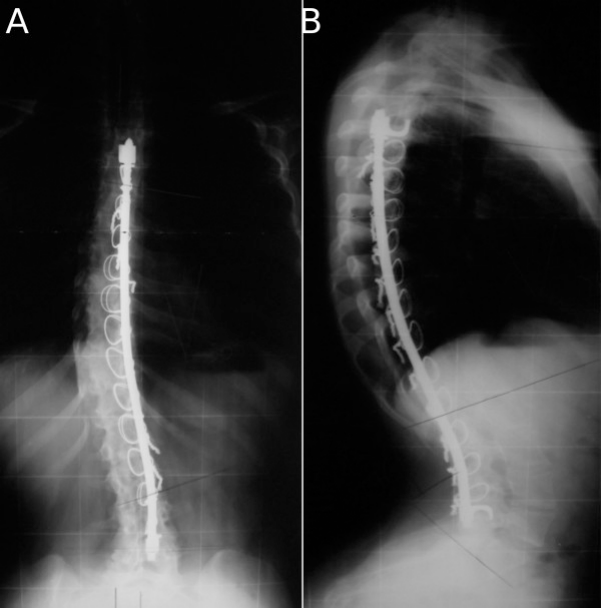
(A, B), Case 1: Spine radiographs at a 9-year follow-up; anteroposterior (A) and laterolateral (B) view.
